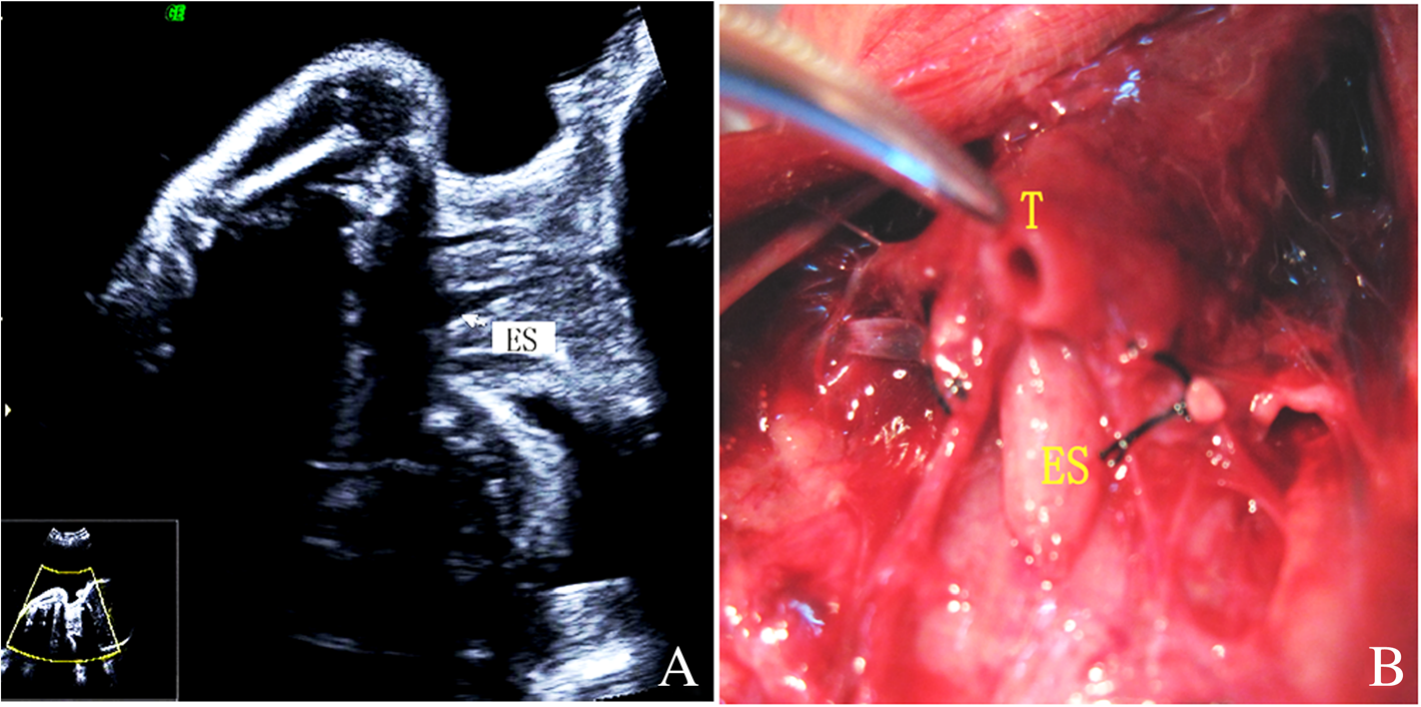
A 22.3-week gestational age fetus with levocardia and esophageal atresia. A cystic dilated esophagus was observed during fetal ultrasound screening (a), and autopsy confirmed esophageal atresia (b)
